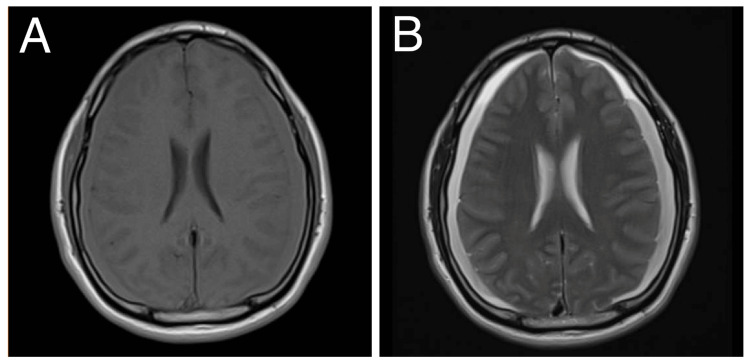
Non-contrast cranial MRI in (A) T1 and (B) T2 axial views showing a hyperacute to acute subdural hemorrhage along both cerebral convexities.
